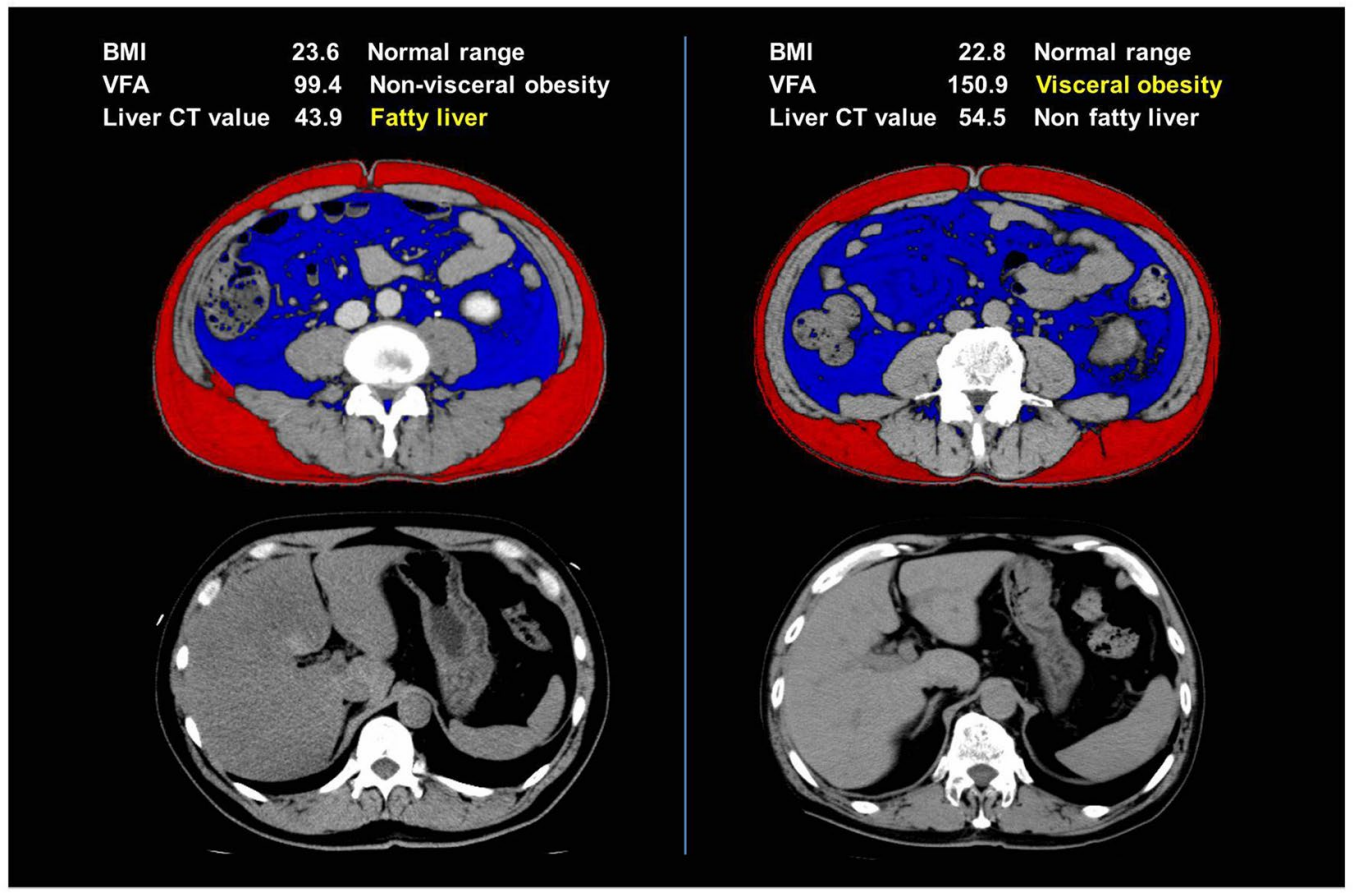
CT images of two representative RCC patients in the normal BMI range. BMI = body mass index (kg/m^2^), VFA = visceral fat area (cm^2^), Liver CT value = liver computed tomography value (Hounsfield units).
